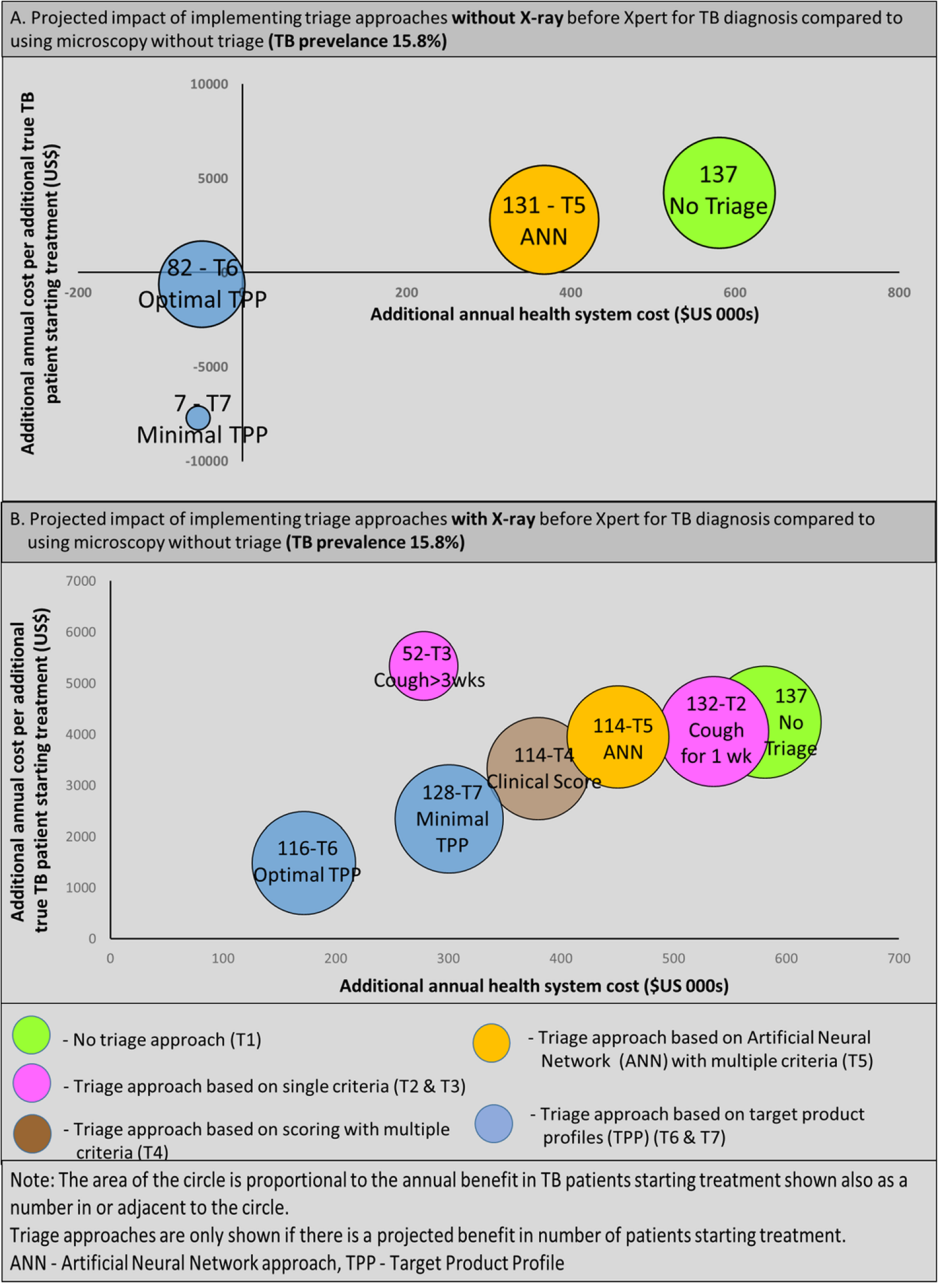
Projections on the impacts of implementing alternative triage approaches (T2-T7) Impacts shown are on health system costs (X-axis), additional cost per additional TB patient starting treatment (Y-axis) and number of additional TB patients starting treatment (size of circle). Graph A is impact of triage without X-ray. Graph B is impact of triage with X-ray
